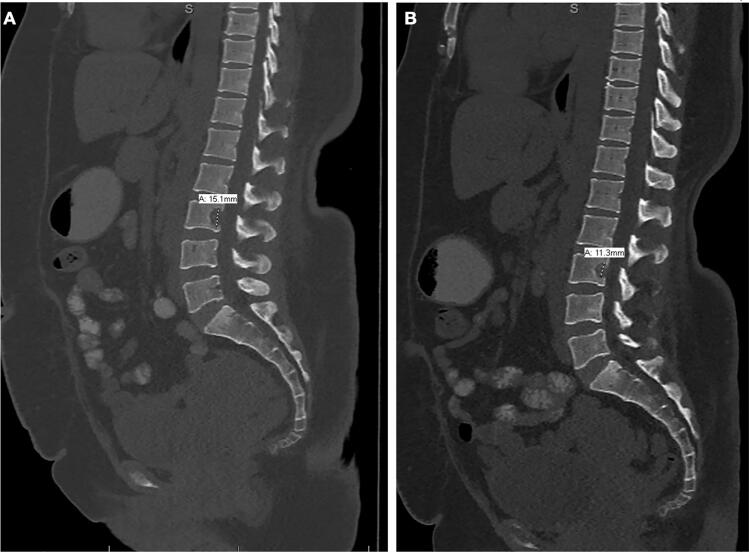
CT scan showing a slight increase in the size of the L3 lesion, measuring 1.5 cm (A), compared to 1.1 cm previously (B).
